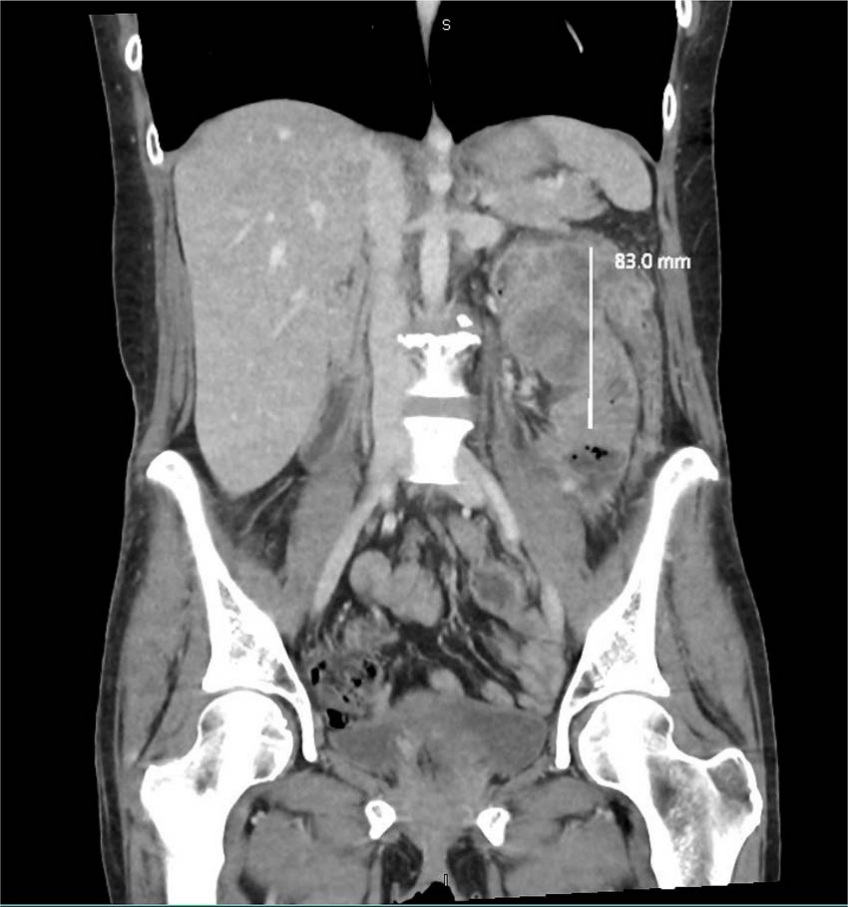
Coronal slice of a CT demonstrating necrotic mass arising from the large bowel with significant wall thickening.
